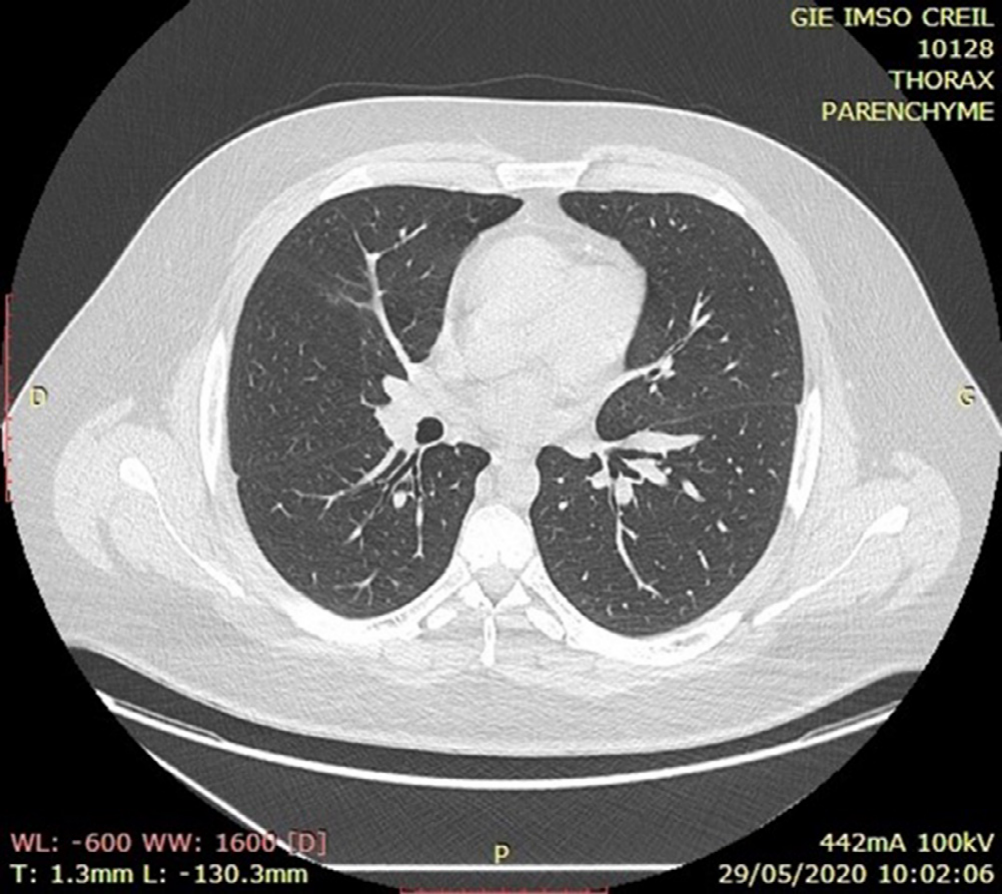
Chest CT-scan at D60: disappearance of lung lesions.
